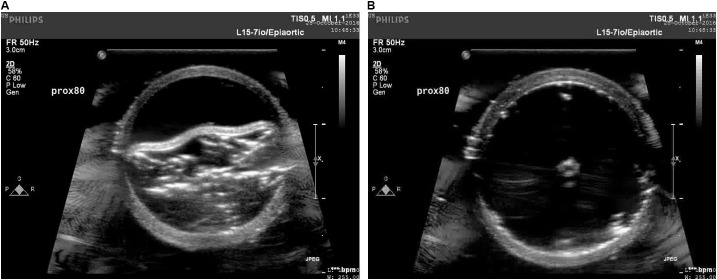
Ultrasound images capturing various stages: (A) crimped and (B) expanded, of a CODA balloon under aortic pressure of 80 mmHg.
